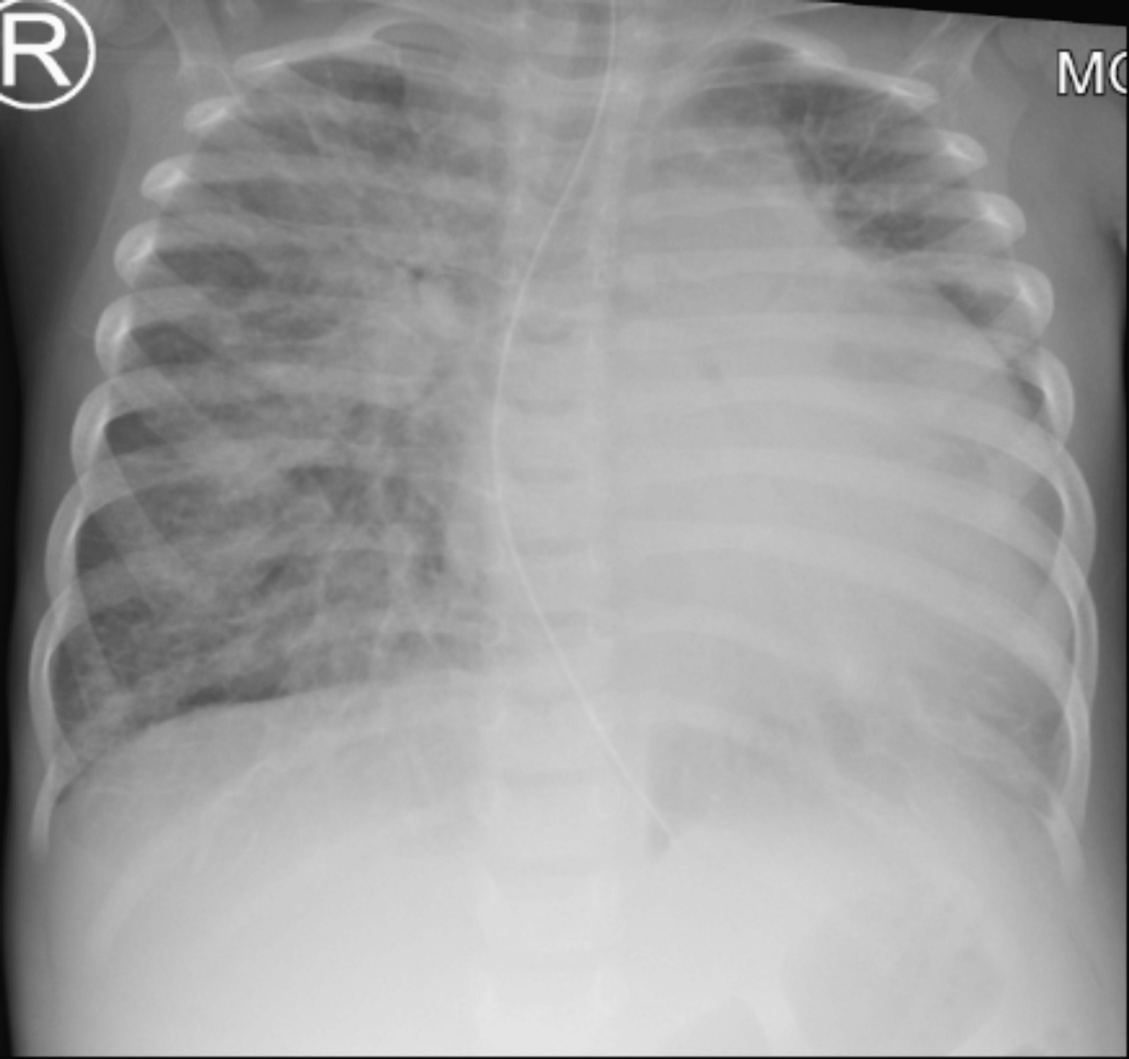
Chest X-ray at day five of admission shows cardiomegaly and pulmonary edema
